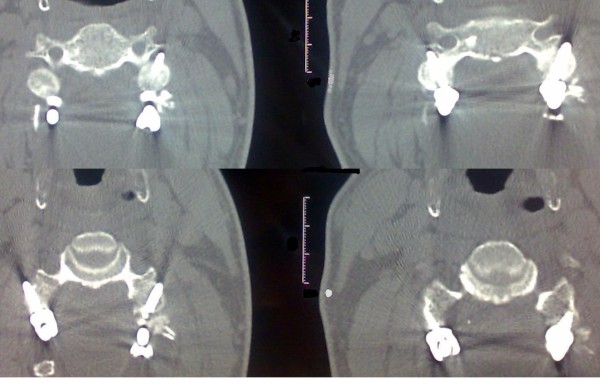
Postoperative axial CT scan slice of C5 showing typical bicorticate screw place in the body of the lateral mass with a 14 mm length. The vertebral foramen is seen not violated. Some bony fusion is also observed (arrow)
